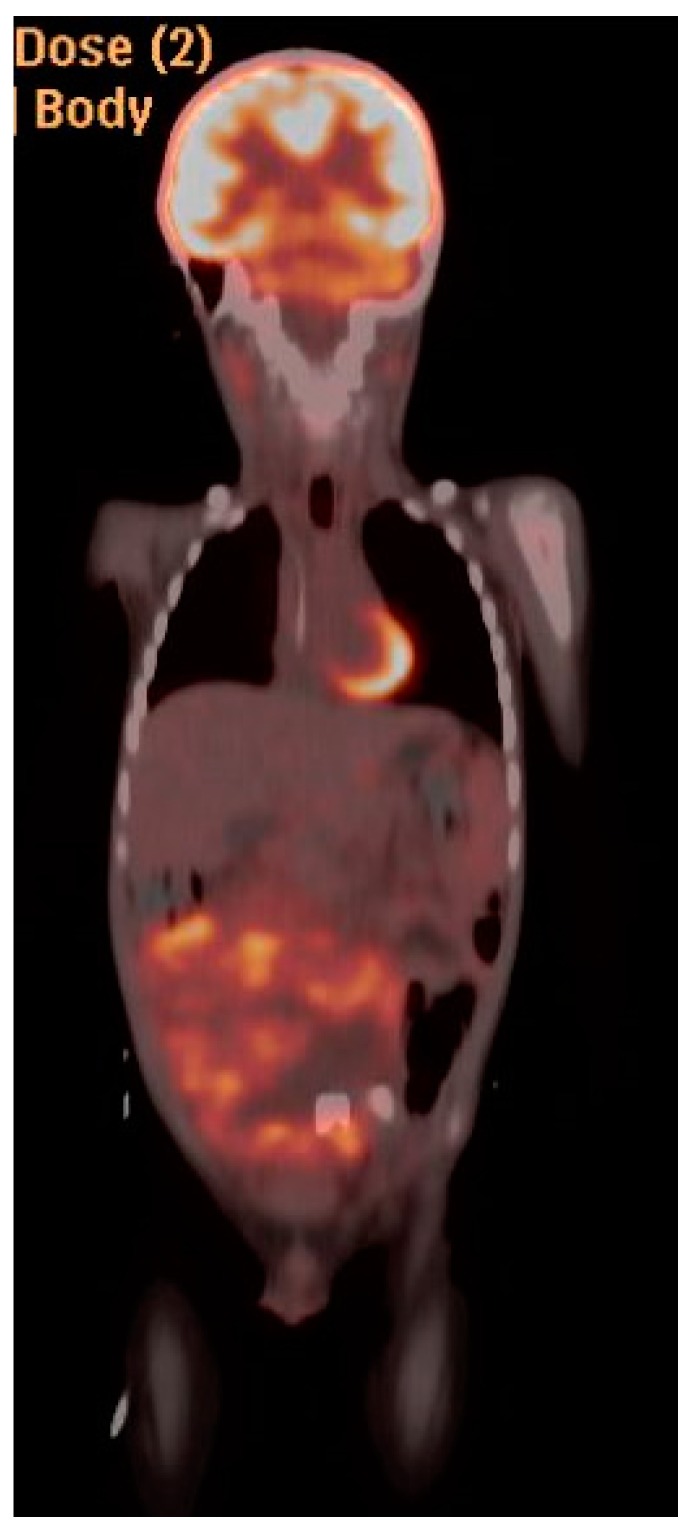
PET CT coronal view of intraabdominal Ewing sarcoma.
